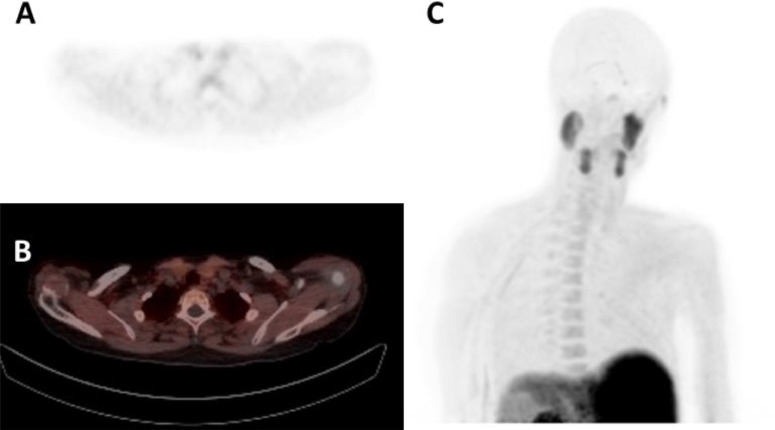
FCH-PET-CT of patient 2. Transversal PET (A) and fused PET-CT (B) images show no increased uptake at this lesion. The MIP (C) shows physiological FCH uptake, no increased uptake at the parathyroid glands.
